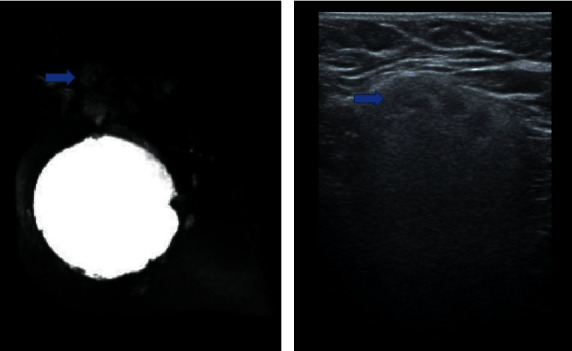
(a and b). 62-year-old women with silicone for 11 years. The MRI (a) shows intact silicone implant in the right breast associated with siliconomas in axillary lymph nodes. The axillary ultrasound (b) confirms the siliconoma as a mass with snowstorm artefact.
